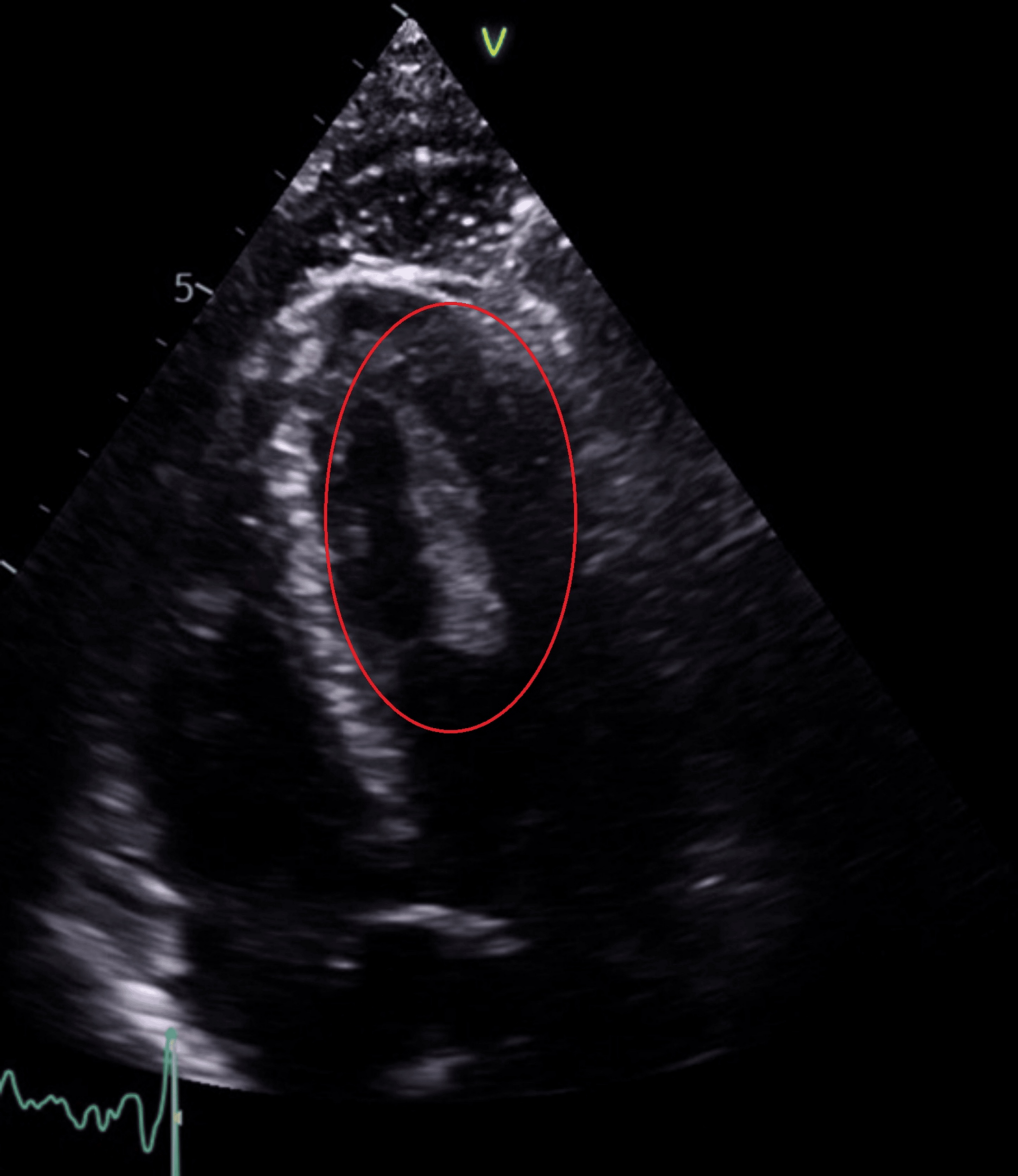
Transthoracic echocardiogram (apical five-chamber view) demonstrating a large, mobile left ventricular (LV) thrombus (red circle) measuring approximately 5.4 × 1.1 cm. The thrombus was attached near the apical region with mild LV systolic dysfunction (estimated EF 45–49%), representing a potential cardioembolic source.
